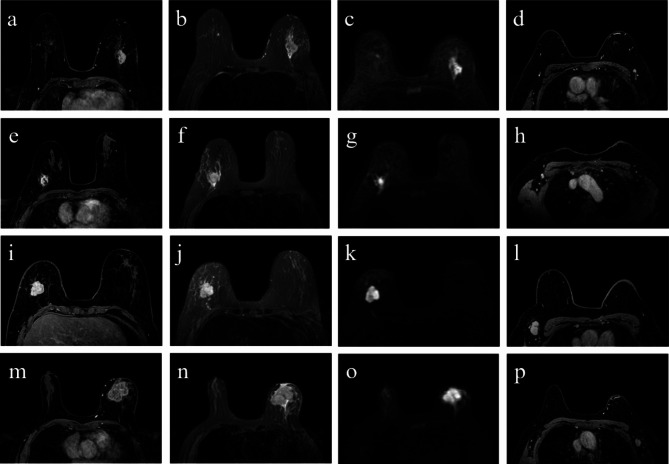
MRI images of four representative cases. (a, e, i, and m) DCE postcontrast phase 1 images of the primary tumor. (b, f, j, and n) T2WI images of the primary tumor. (c, g, k, and o) DWI images of the primary tumor. (d, h, l, and p) DCE postcontrast phase 1 images of ALN. (a–d) A 57-year-old female patient with breast cancer presented with pathologically confirmed left ALNM. The patient was misdiagnosed with NALNM by the junior radiologist but was correctly diagnosed by the senior radiologist and the combined DCE postcontrast phase I + T2WI + DWI model. (e–h) A 66-year-old female patient with breast cancer presented with pathologically confirmed right NALNM. The patient was misdiagnosed with ALNM by the senior radiologist but was correctly diagnosed by the junior radiologist and the combined DCE postcontrast phase I + T2WI + DWI model. (i–l) A 65-year-old female patient with breast cancer presented with pathologically confirmed right NALNM. The patient was misdiagnosed with ALNM by the senior and junior radiologists but was correctly diagnosed by the combined DCE postcontrast phase I + T2WI + DWI model. (m–p) A 59-year-old female patient with breast cancer who presented with pathologically confirmed left ALNM. The patient was correctly diagnosed by the senior and junior radiologists and the combined DCE postcontrast phase I + T2WI + DWI model
